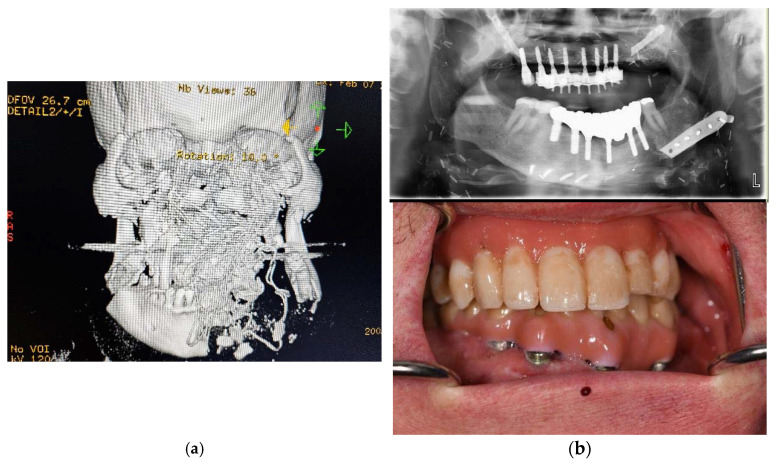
Primary computed tomography (CT) of patient no. 6 upon arrival (a). Most of the maxilla and nasal structures are missing, the outer frame of the zygomatic bones have comminuted fracture, and the mandible has comminuted fracture as well as some missing parts. The bleeding was tamponated with cloth, and the radio-opague marking of the tissue can be seen in the 3D reconstruction. The missing bone in the maxilla and mandible of the patient no. 6 had been reconstructed earlier with vascularized bone using microvascular free flap positioned in the desired vertical and horizontal position using virtual planning and CAM-produced osteosynthesis (Planmeca Promodel, Helsinki, Finland). The custom osteosynthesis from the reconstructed maxilla had been mostly removed for dental reconstruction (upper b). The final dental rehabilitation involved a two-in-one structure in the maxilla and a conventional implant bridge in the mandible (lower b).
